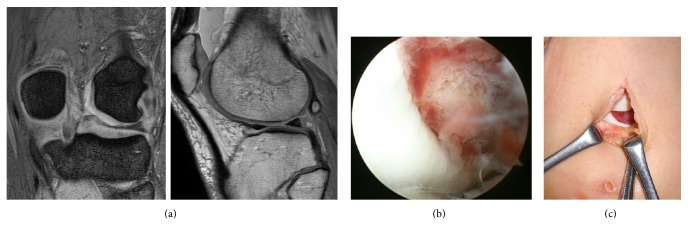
Findings in Patient 1. (a) MRI, showing an osteochondral defect in the lateral femoral condyle. (b) Arthroscopy, showing a 2 × 1.5-cm osteochondral defect in the lateral femoral condyle. (c) Arthrotomy was applied, fixing the osteochondral fragment.
